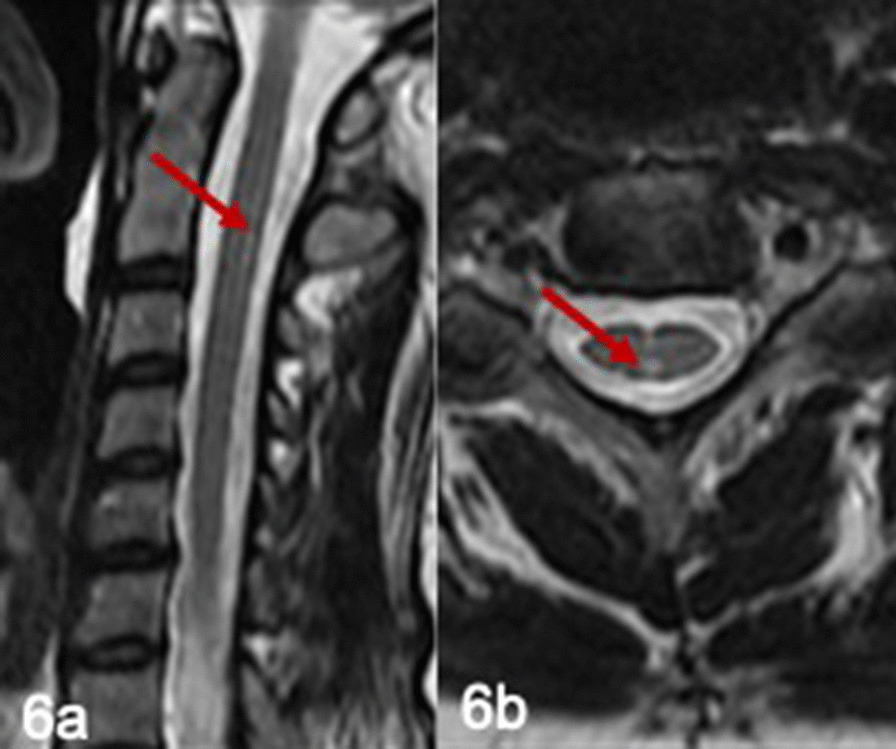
Spinal MRI in patient 4. T2 weighted sagittal (a) and axial view (b) shows a focus of high signal posteriorly in the cervical spine (arrows)
